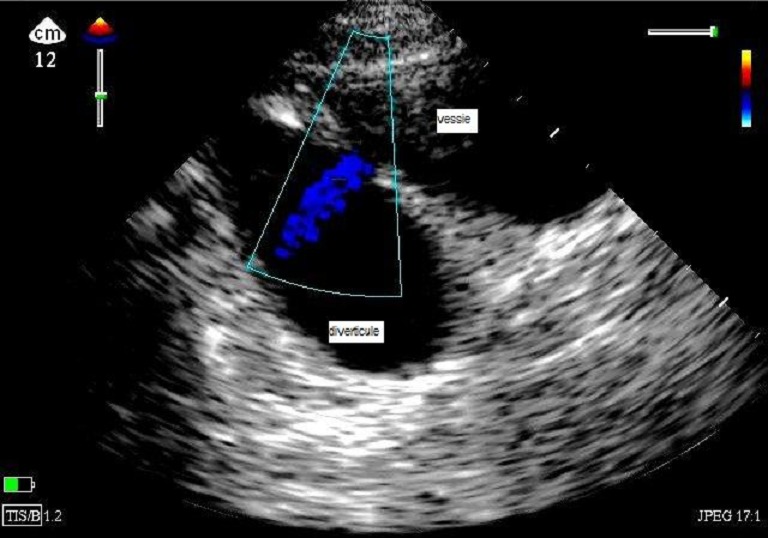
Image échographique mode 2 D avec doppler couleur montrant le passage des urines à travers le diverticule après pression dosée sur une vessie pleine chez un enfant de 13 ans
